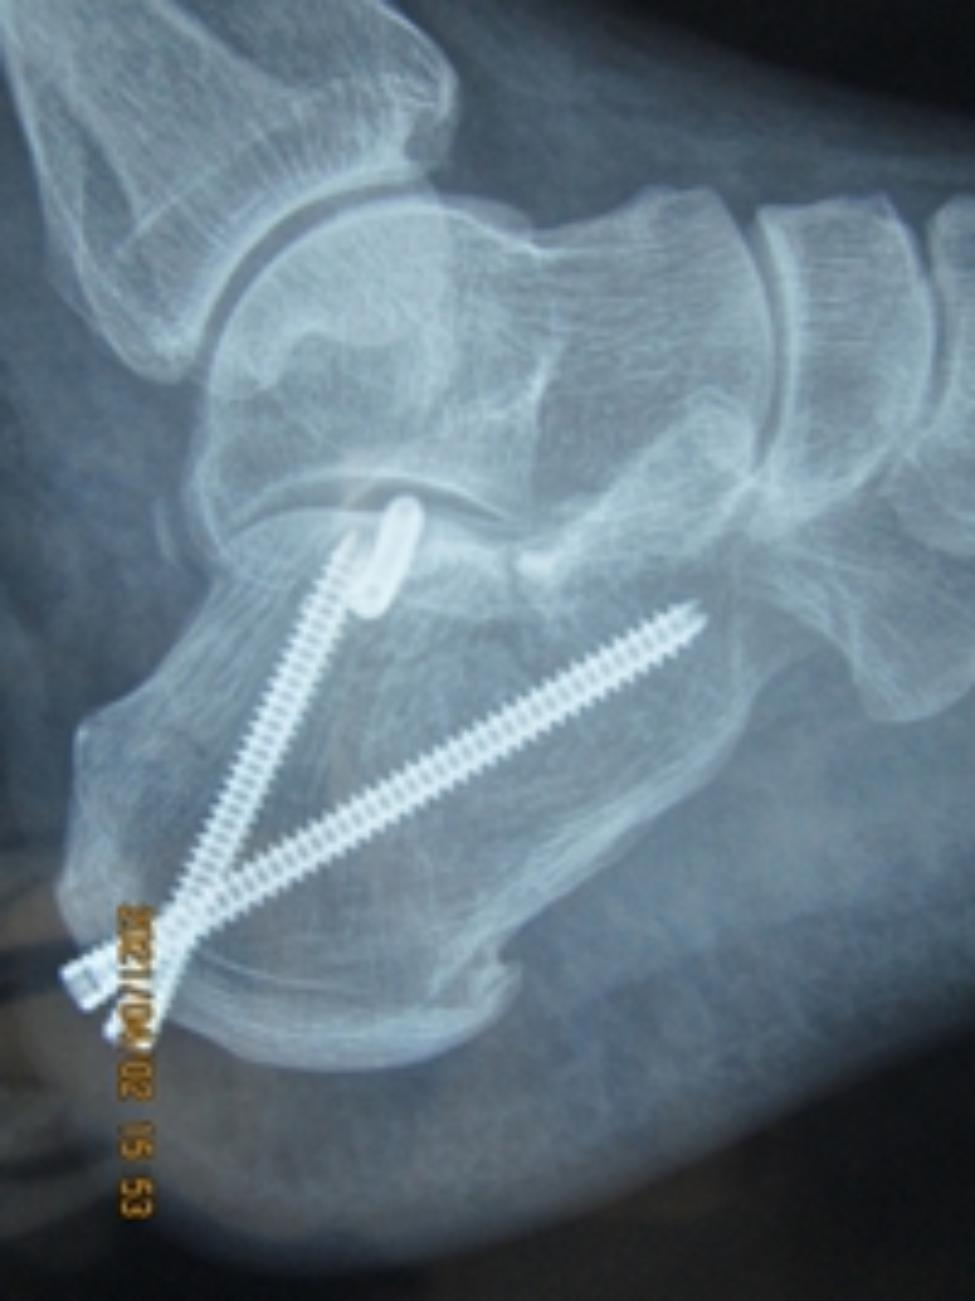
X-ray of incorrect screw placement affected subtalar joint
